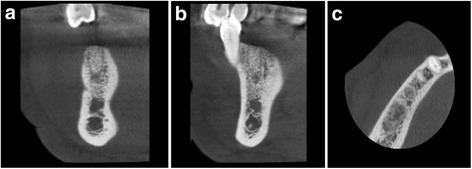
a–c Four-month postoperative CBCT showing graft integration and preservation of ridge without collapse of the buccal or lingual cortical plates also showing the cross sections in the grafted area
